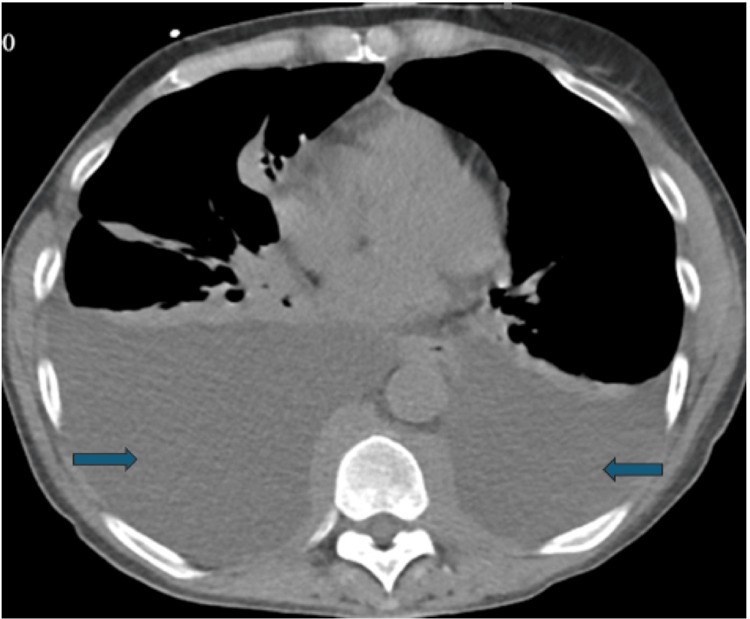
Axial CT of the thorax demonstrating bilateral pleural effusions (arrows), more pronounced on the right, with associated compressive atelectasis of the lower lobes.
